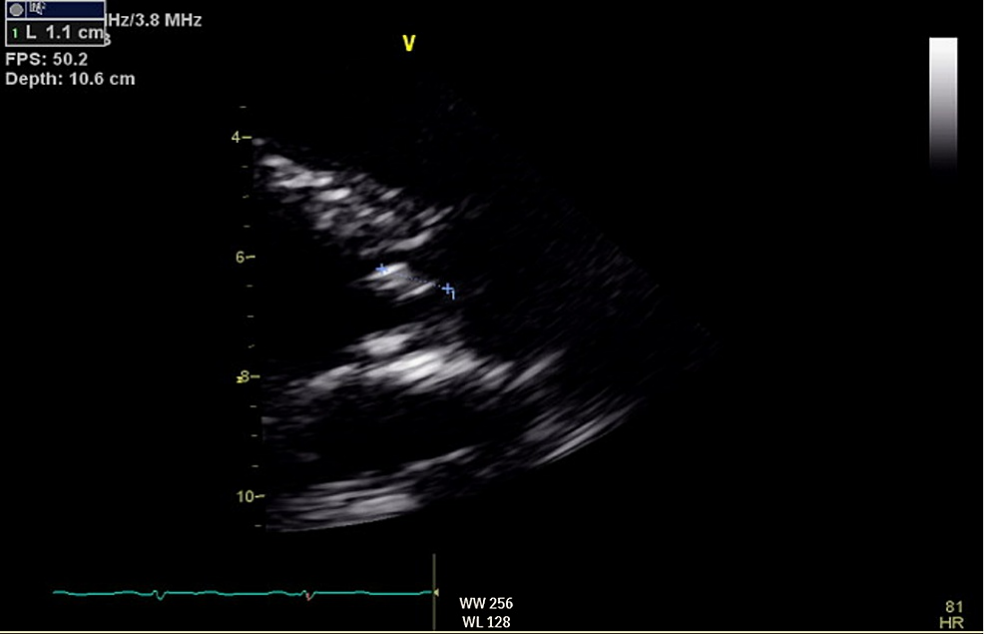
PLAX view-vegetation measuring 1.1 cm on the right coronary cusp. PLAX: parasternal long-axis view.
